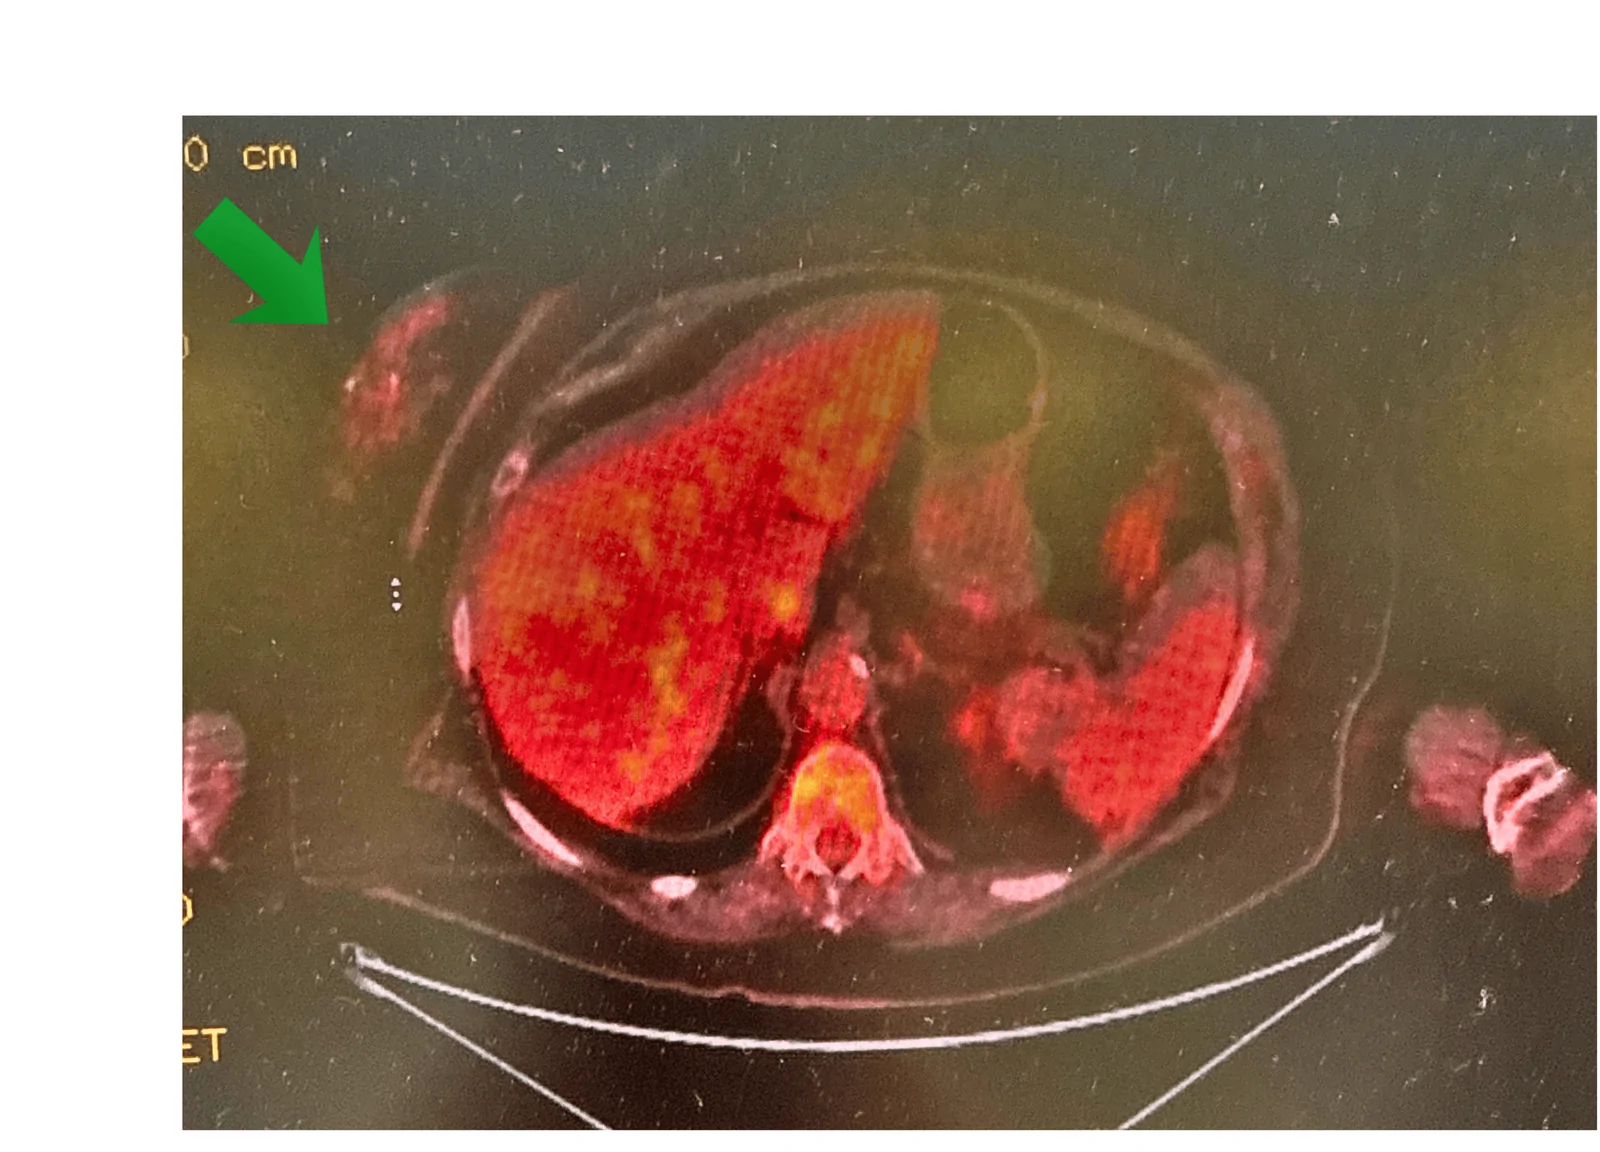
18F-FDG PET/CT demonstrating right breast and axillary disease Axial fused PET/CT image demonstrates FDG-avid right-sided breast and regional axillary disease, corresponding to the subsequently biopsied high-grade neuroendocrine carcinoma. 18F-FDG: fluorine-18 fluorodeoxyglucose, PET/CT: positron emission tomography/computed tomography
